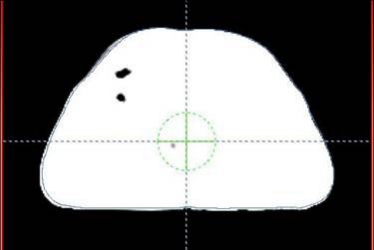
Contour mismatch when CT contrast is adjusted.
